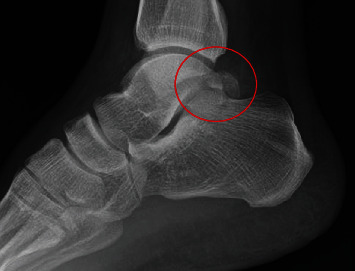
Lateral radiograph of right ankle demonstrating a prominent fractured and displaced posterior process of talus.
